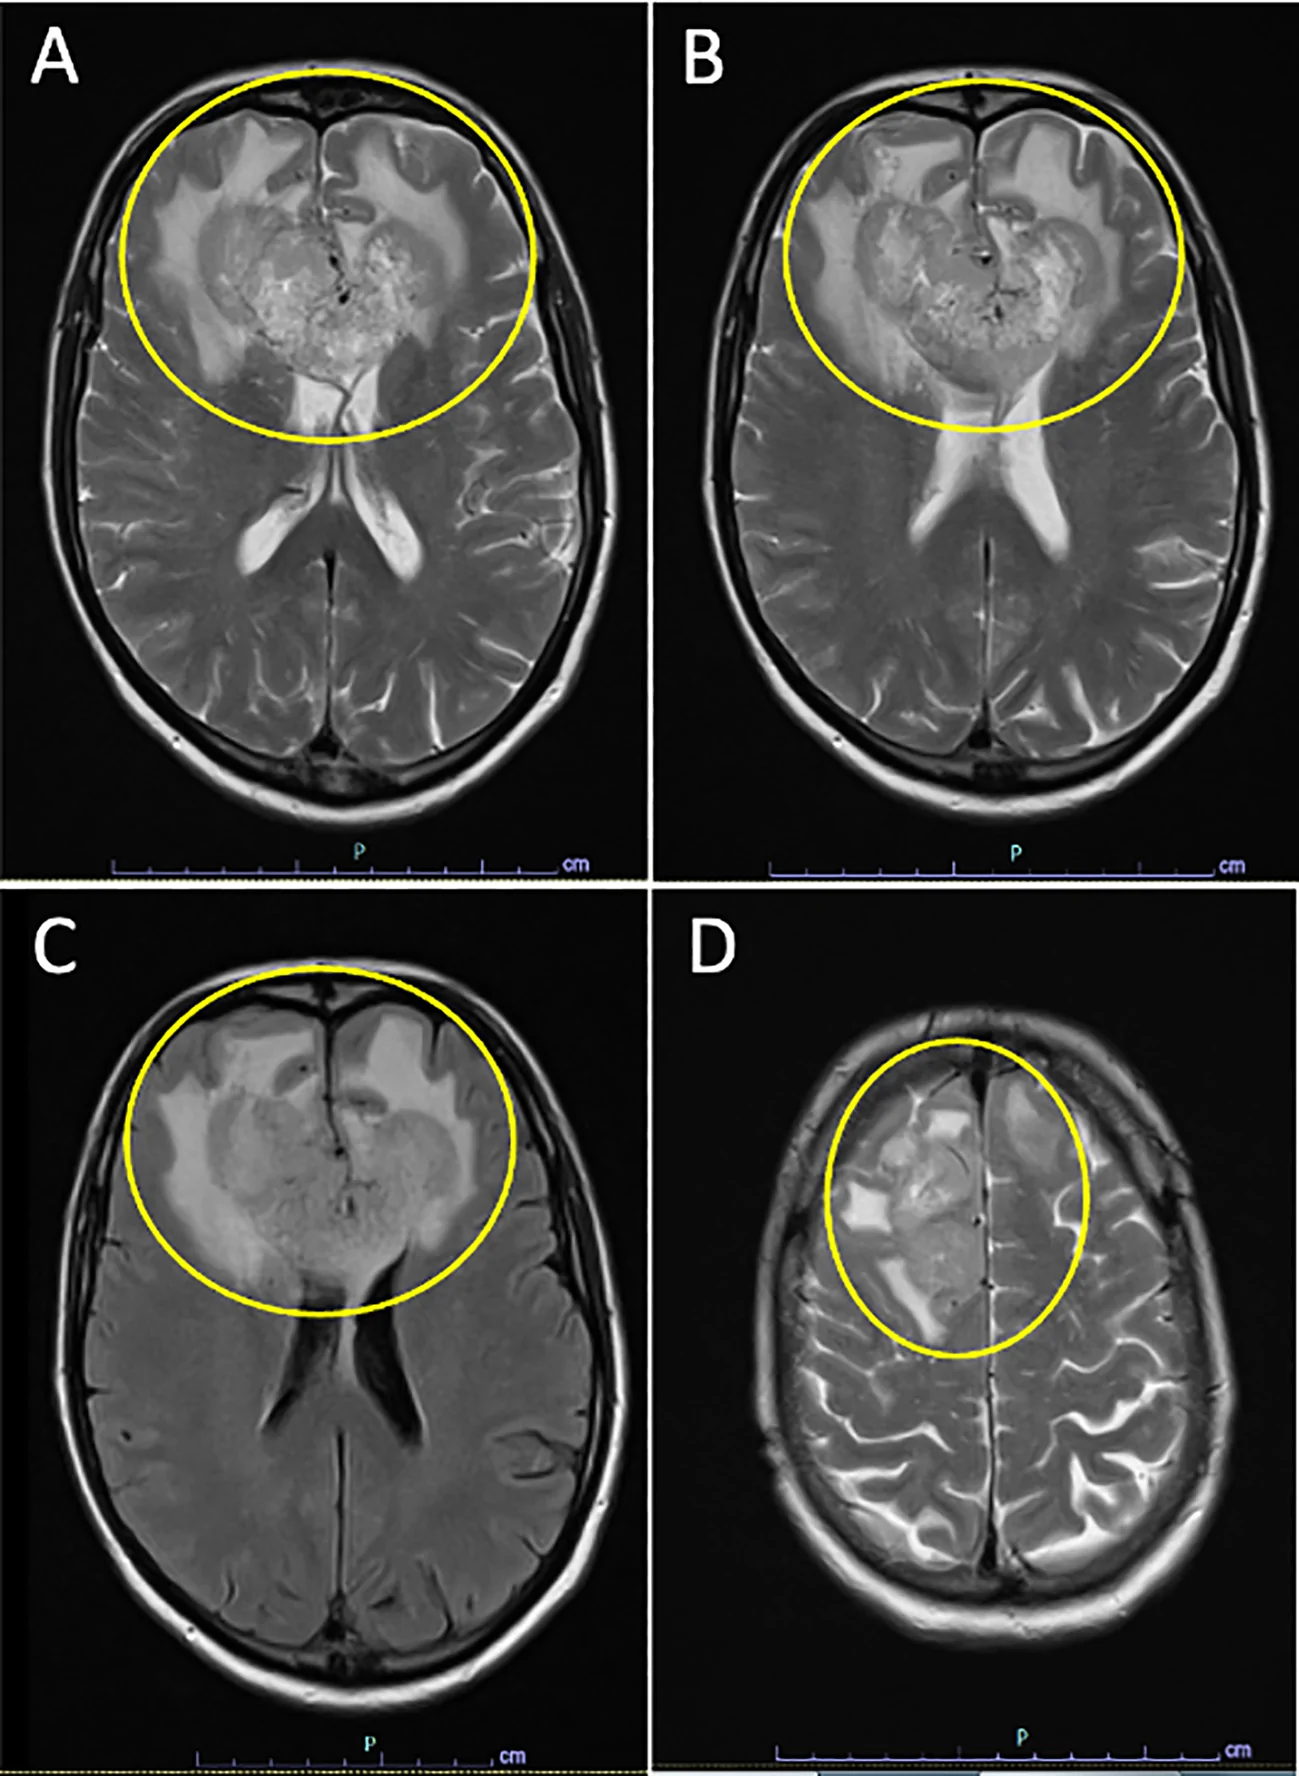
Cranial MRI (A–D) showing multifocal GBM with the main component centered at the genu of the corpus callosum and involving both frontal lobes, with an additional lesion in the right superior frontal gyrus. Yellow circles indicate the lesions.
